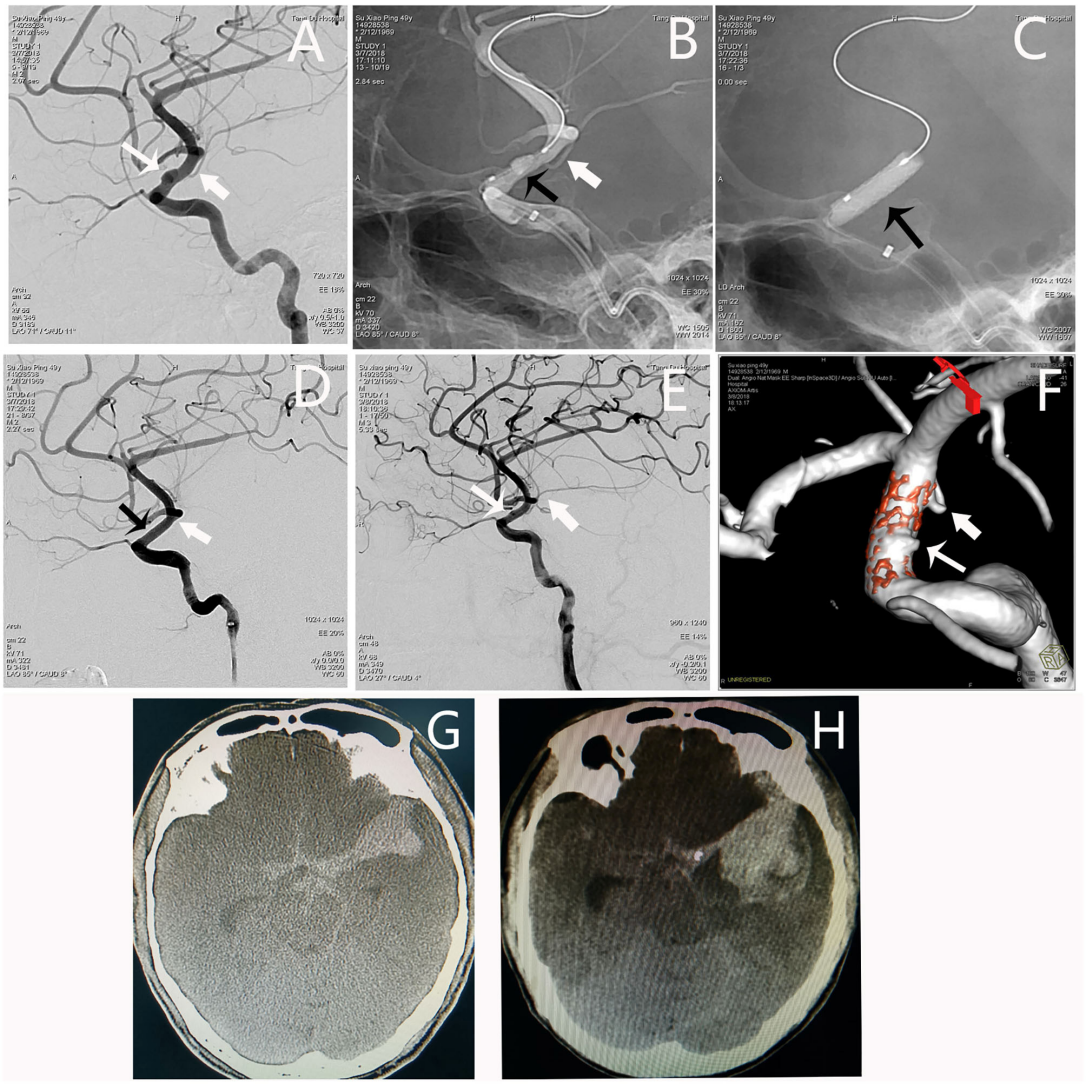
Angiographic images of a 49-year-old man (Patient no. 13 in Table 1) with a L-ICA C6 BBA. (A) A BBA (1.8*5.1 mm) located on the lateral wall of the C6 segment (white thin arrow); the AHCA was indicated by the white fat arrow. (B,C) A 3.5*10-mm WCS was deployed (black arrow). (D) Immediate postoperative angiography showing the aneurysm and the ACHA were both occluded. (E,F) DSA and dual volume technology showing aneurysm recurrence, and the AChA appeared again. (G) Preoperative CT scan showing subarachnoid hemorrhage. (H) The CT scan showing significantly increased hematoma as the patient fell in coma.
